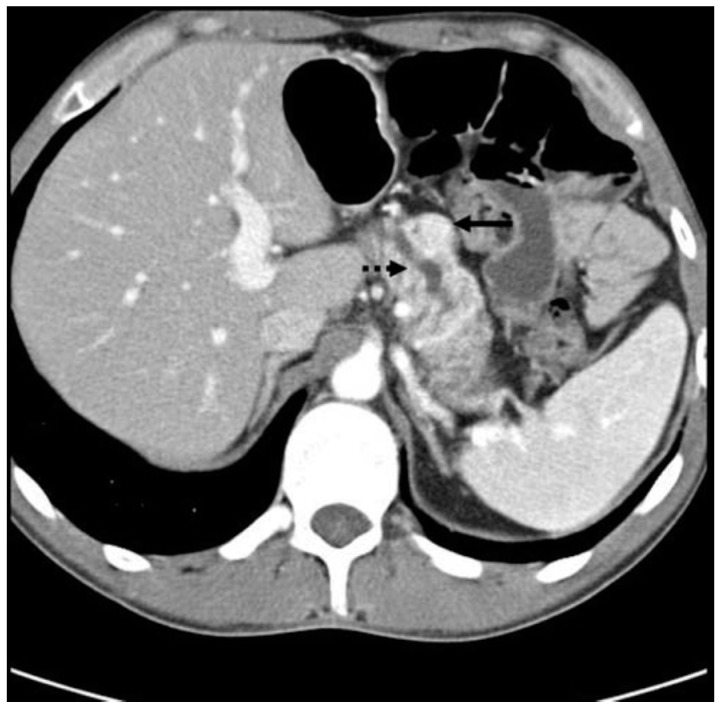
CT scan showing a PPoma of the head (solid arrow) of the pancreas causing pancreatic duct dilation (dashed arrow). The tumor is a discrete lesion, with well-defined borders, and shows contrast enhancement in the arterial phase [30].
